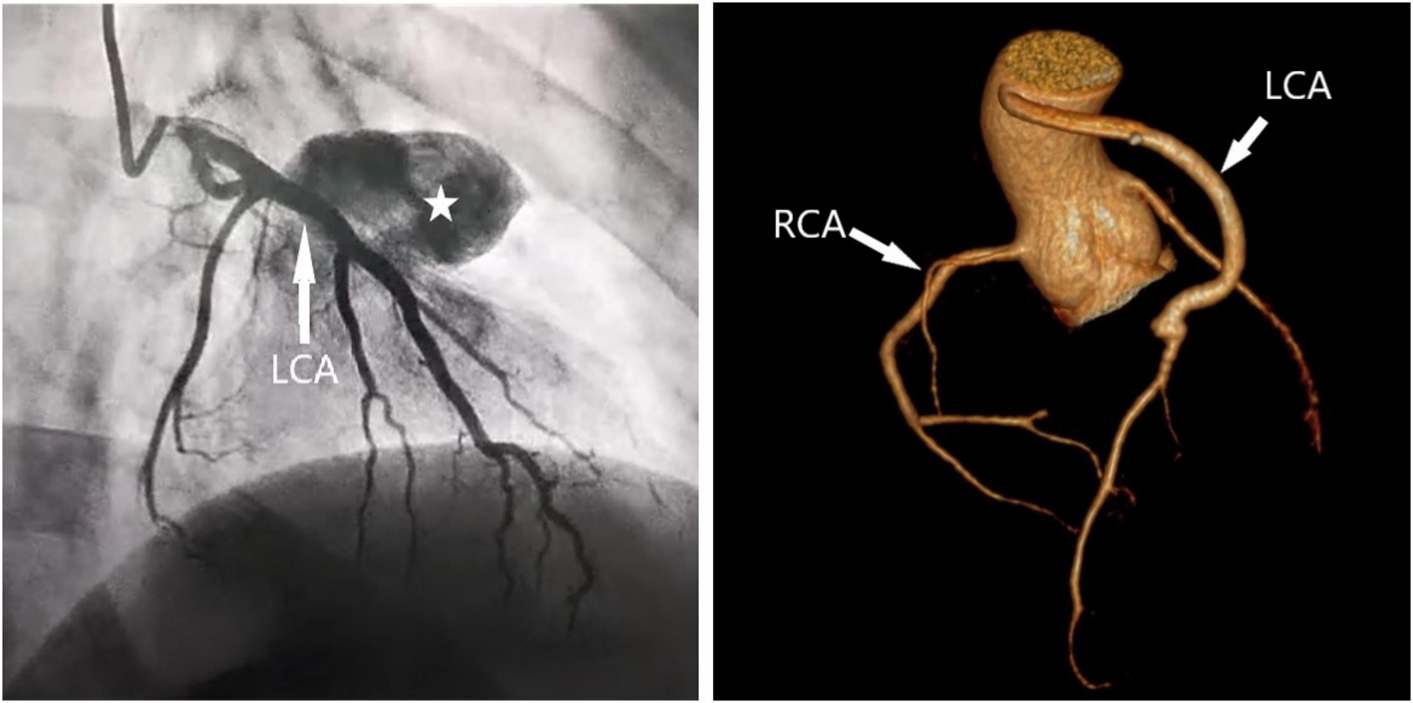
Preoperative coronary angiography and Postoperative cardiac CTA. (A) Preoperative coronary angiography showed a giant coronary aneurysm in the left anterior descending artery. (B) Post-operative CTA showed a favorable configuration of the left coronary artery.
